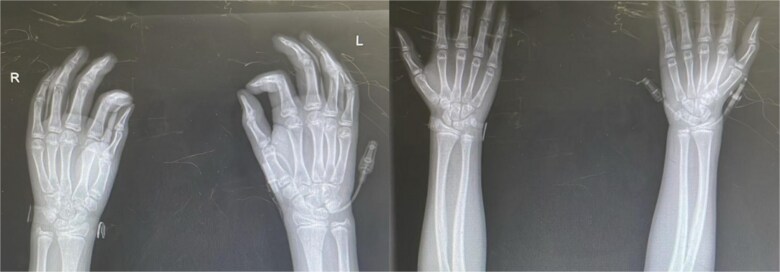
Hands (X-ray). Radiographic (X-ray) evidence shows advanced skeletal development, with phalangeal epiphyses appearing more developed than expected for a 5-year-old. This is consistent with the patient’s accelerated bone age, which is a key indicator of precocious puberty.
